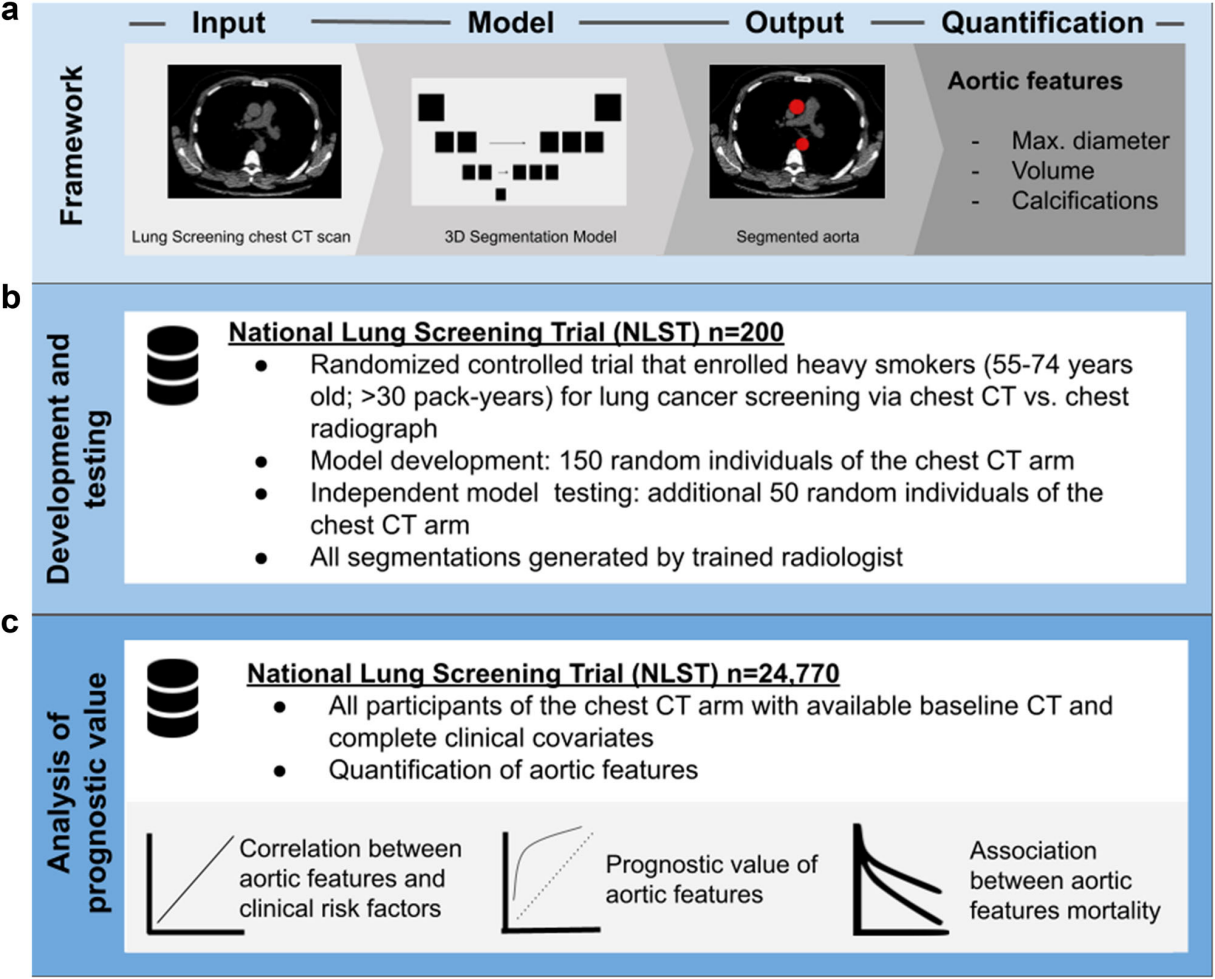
Overview of the study design. a The framework was developed to automatically quantify features of aortic disease defined as 1) maximum diameter, 2) volume and 3) calcifications on non-contrast-enhanced lung screening chest CT. b For development, a random sample of 150 NLST participants was used. Independent testing was performed in an additional random sample of 50 participants not seen during development. c The prognostic value of the aortic features was investigated in 24,770 NLST participants. CT computed tomography, NLST National lung screening trial.
